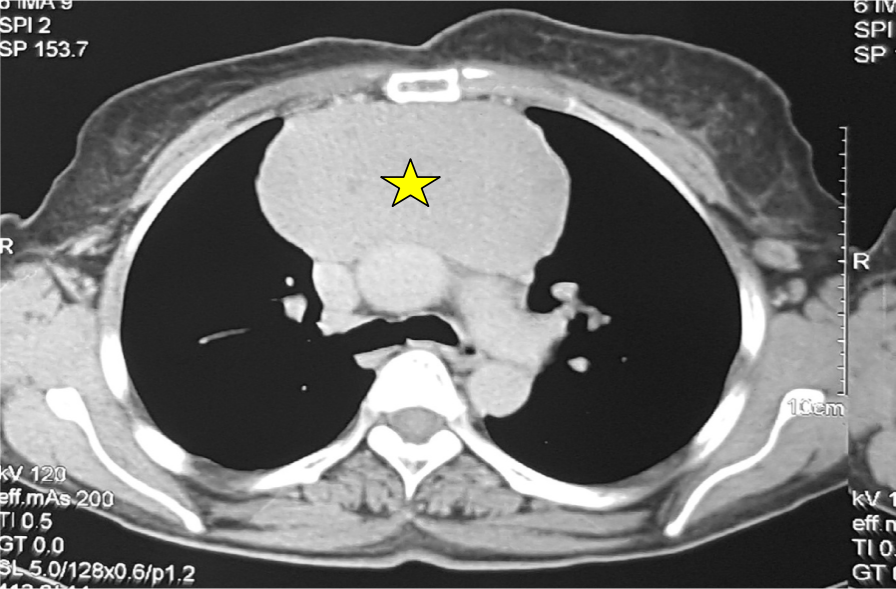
Chest CT showing an anterosuperior mediastinal mass (the yellow star represents the tumor mass)
